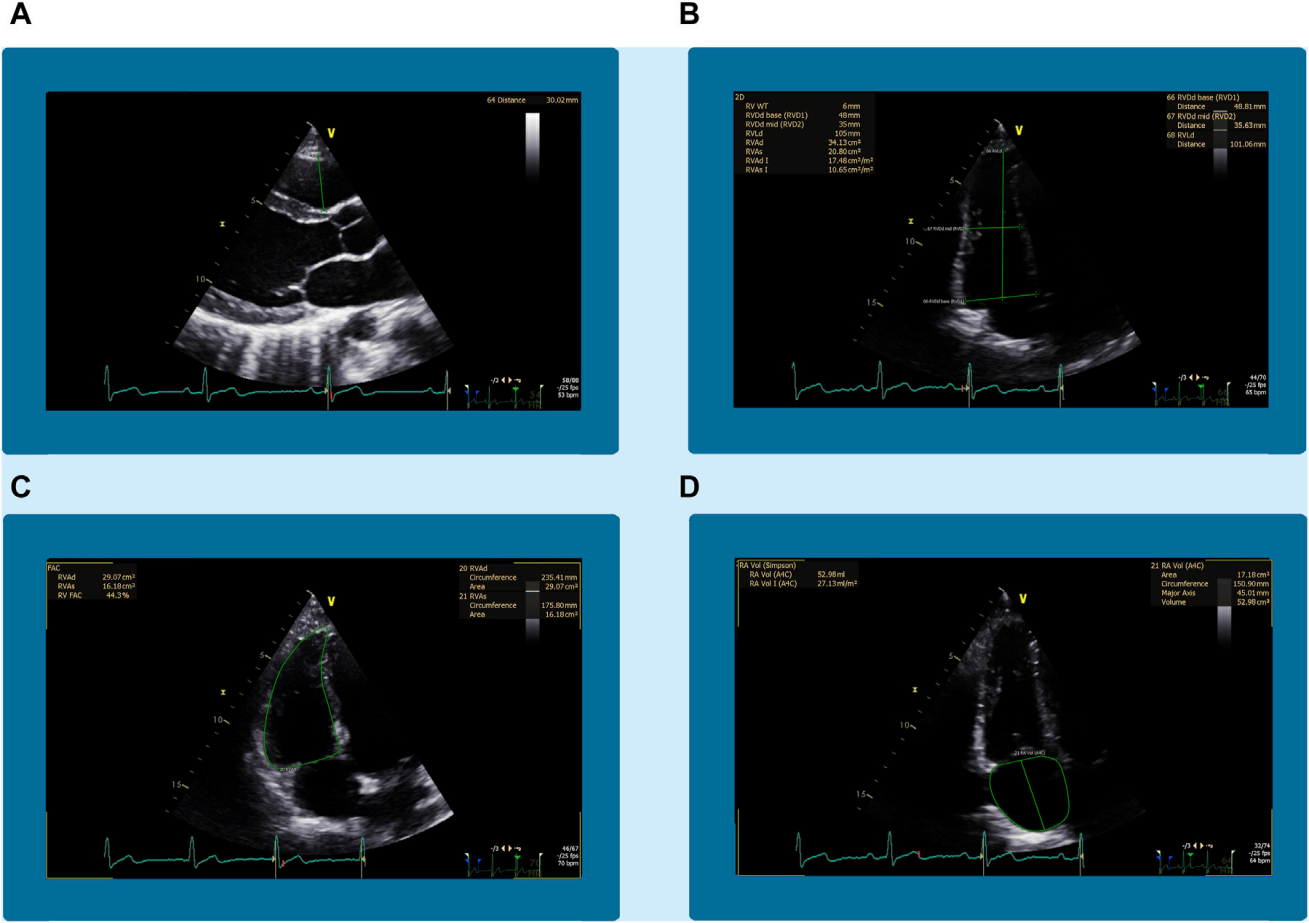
An example of the echocardiographic measurements from the right heart. Panel (A) shows the right ventricular outflow tract (RVOT) measured in the parasternal long-axis view. Panel (B) shows the basal (RVD1), mid-cavity (RVD2), and longitudinal (RVD3) diameters of the right ventricle (RV) in the four-chamber view of the right heart. Panel (C) shows the same view of the right ventricular end-diastolic area (RVEDA). Panel (D) shows the right atrial volume (RAV) in the four-chamber view focused on the right heart.
